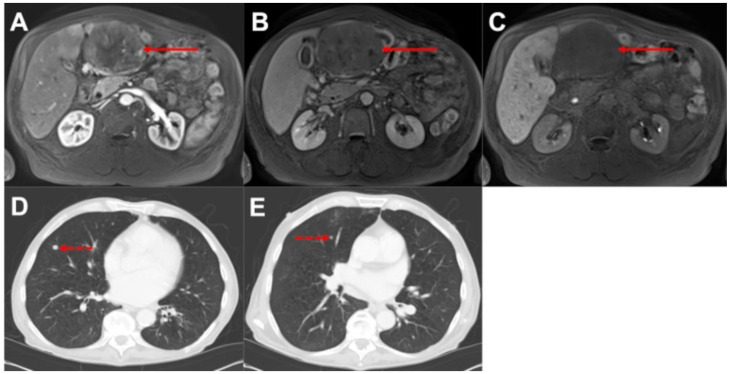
No change in management despite CT-detected EHD. (A): liver MRI, arterial phase, and axial plane. (B): liver MRI, venous/transitional phase, and axial plane. (C): liver MRI, hepatobiliary phase, and axial plane. (D,E): chest CT, lung window, and axial plane. Note the solitary, typical HCC in liver segment III ((A–C), solid arrows) demonstrating arterial hypervascularization (A), portalvenous phase washout (B), and hypointensity in the hepatobiliary phase (C). CT showed two additional pulmonary metastases in the right lung ((D,E), dashed arrows). However, EHD did not lead to a change of the therapy recommendation, as palliative treatment was already recommended due to close proximity of the primary tumor to central vascular structures.
